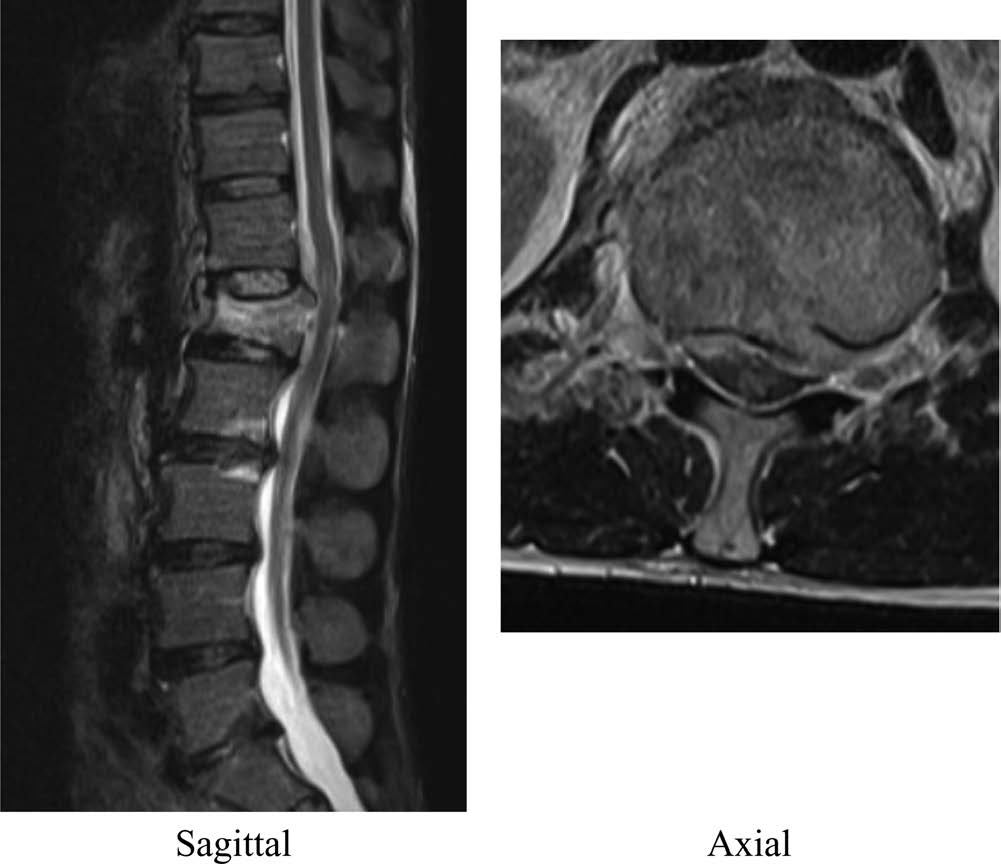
Magnetic resonance imaging showed significant compression of the conus and intramedullary signal changes.
